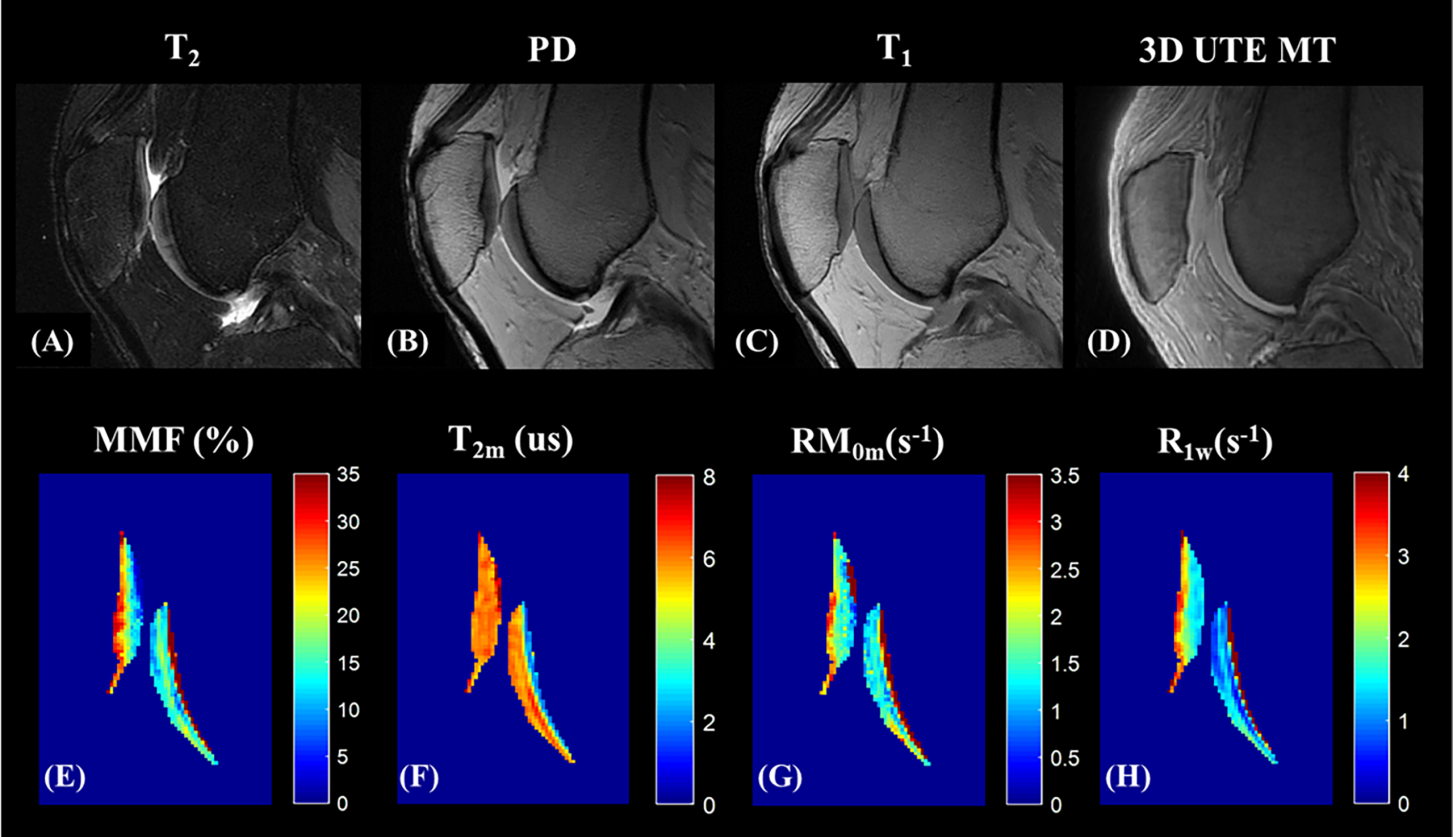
UTE-MT modeling (mapping) of knee cartilage. Panels (A–D) show the representative *ex vivo* knee MR images acquired from clinical sequences (A–C) and 3D UTE -MT (MT flip angle of 500° and frequency offset of 50 KHz) (D). Color mapping of UTE-MT modeling parameters, including MMF (%) (E), T_2_ relaxation time of the macromolecular pool (T_2m_, us) (F), proton exchange rate from water to macromolecular pool (RM_0m_, s^-1^) (G), and spin-lattice relaxation rate of the water pool (R_1w_,s^-1^) (H) are demonstrated.
